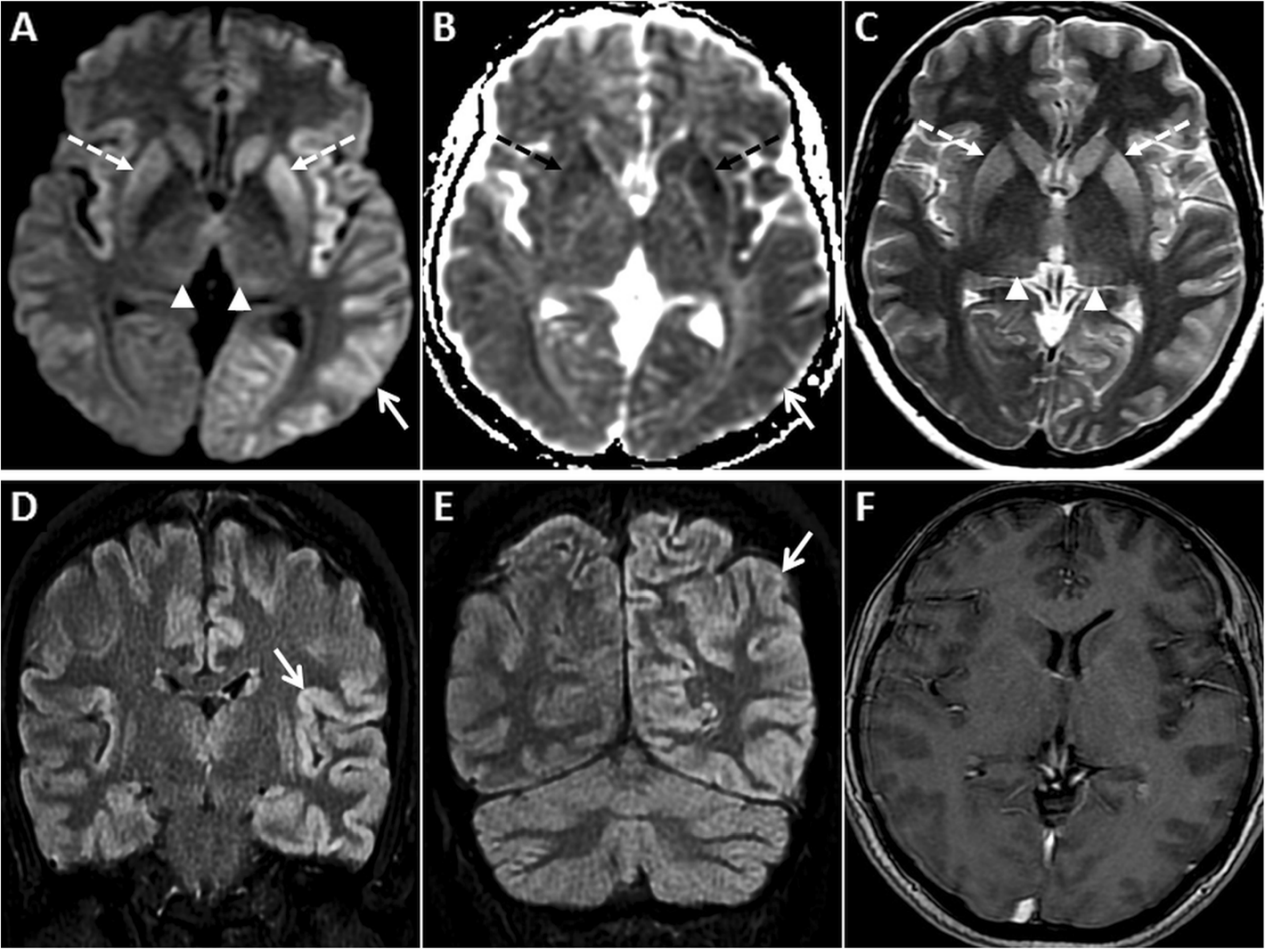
Pre- and post-contrast MRI of the brain in a 55-year-old male patient who presented with rapidly progressive dementia due to sCJD. GRD is seen in the left temporo-parieto-occipital region (arrows) and in the insular regions on axial DWI (a) and ADC image (b). Nearly symmetric restricted diffusion is also seen in the basal ganglia (dashed arrows) along with mild DWI hyperintensity in the pulvinar nuclei (arrowheads). Axial T2W image (c) shows T2 hyperintensity in the basal ganglia (dashed arrows) and pulvinar nuclei (arrowheads). Sequential anteroposterior FLAIR images (d, e) demonstrate the extent of the cortical signal abnormality (arrows). No enhancement is seen in these regions on axial post-contrast T1W image (f)
